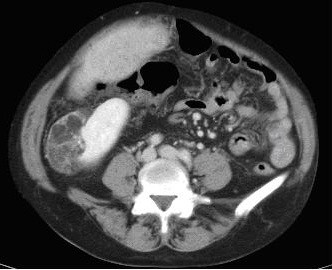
Axial CT image through the abdomen demonstrates a well-defined mass arising from the lower pole of the right kidney containing fat and soft tissue elements consistent with an angiomyolipoma.
